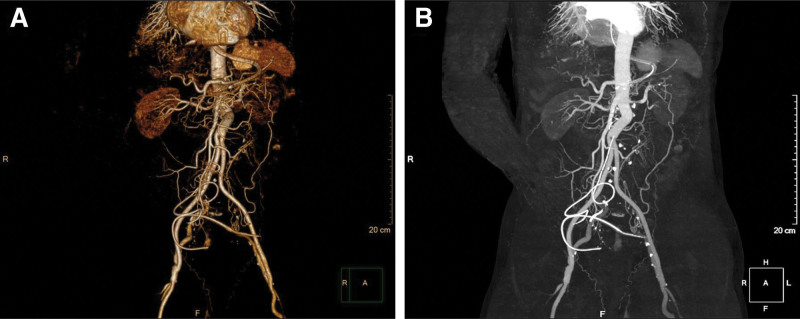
Successful revascularization achieved through a combination of aorto-bifemoral bypass, bilateral lower extremity thrombectomy, and construction of a femoral-to-distal SFA bypass. (A) Volume rendering of CTA; (B) Maximum intensity projection of CTA. SFA = superficial femoral artery, CTA = computed tomography angiography.
